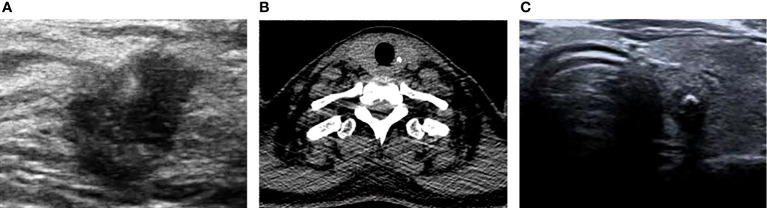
The Doppler ultrasound test of the breast and axilla demonstrated a large, 1.85 × 1.12 × 1.54 cm   retro-areolar lump at the 8 o’clock position (A). The CT scan of the thyroid presented a 4-mm calcium lesion in the left lobular (B). The Doppler ultrasound test of the thyroid gland showed a large, 0.65 × 0.58 × 0.65 cm TI-RADS 4a solid nodule with calcification found in the middle-upper part of the left lobular (C).
